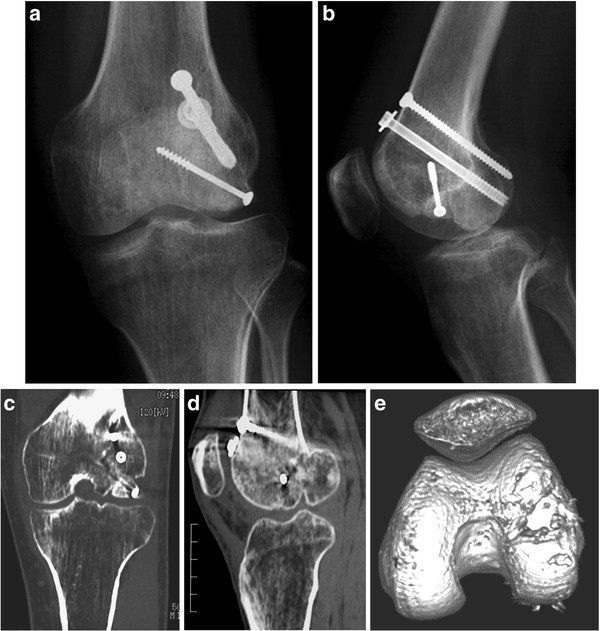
Anteroposterior (a) and lateral (b) radiographs, and computed tomography scans (c-e) of the left knee 4 months after the first operation, showing the malunited lateral femoral condyle. Figure 1d scale bar: 5 cm.
